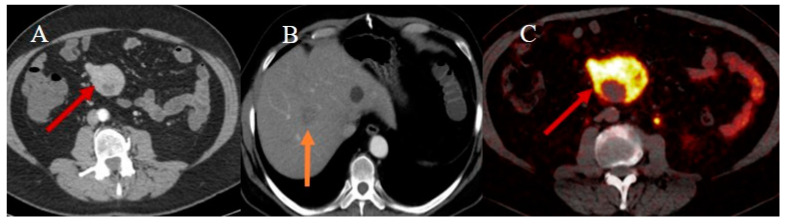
Image (A) displays a contrast-enhanced computed tomography (CECT) image of a 75-year-old patient who came to the emergency room with abdominal pain. A hypervascular ileal mass was discovered (red arrow) during the examination. The patient also presented with liver metastases (orange arrow), one of which is depicted in image (B) (orange arrow). The ileal mass exhibited increased uptake of 68Ga-DOTA PET/CT as visualized in image (C) (red arrow) and was determined to be compatible with an ileal neuroendocrine tumor (NET).
